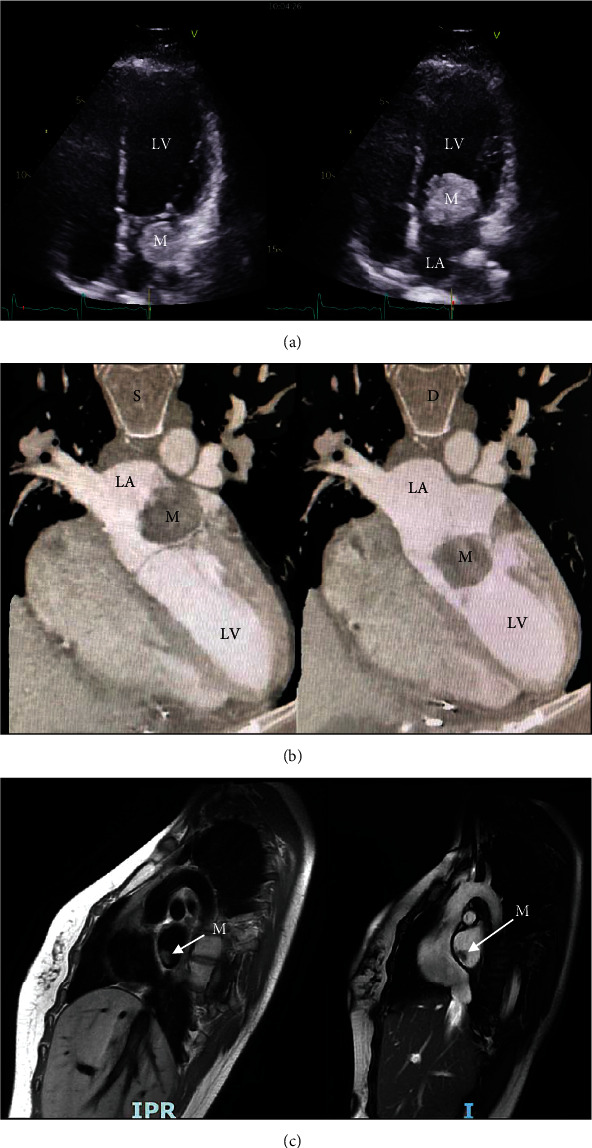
(a) Four-chamber echographic view shows a mobile left atrial mass (M) protruding into the left ventricle (LV) whose ultrasonic characteristics are consistent with a myxoma (LA: left atrium). (b) MRI performed in 2021 shows the mass (M) protruding through the mitral valve from the left atrium (LA) during systole (S) into the left ventricle (LV) during diastole (D). (c) MRI (sagittal T2- and T1-weighted images) performed in 2007 shows the first occurrence of a 17 × 14 mm left atrial mass (M). Video link on YouTube: https://www.youtube.com/watch?v=cPPDHg0cYZc.
